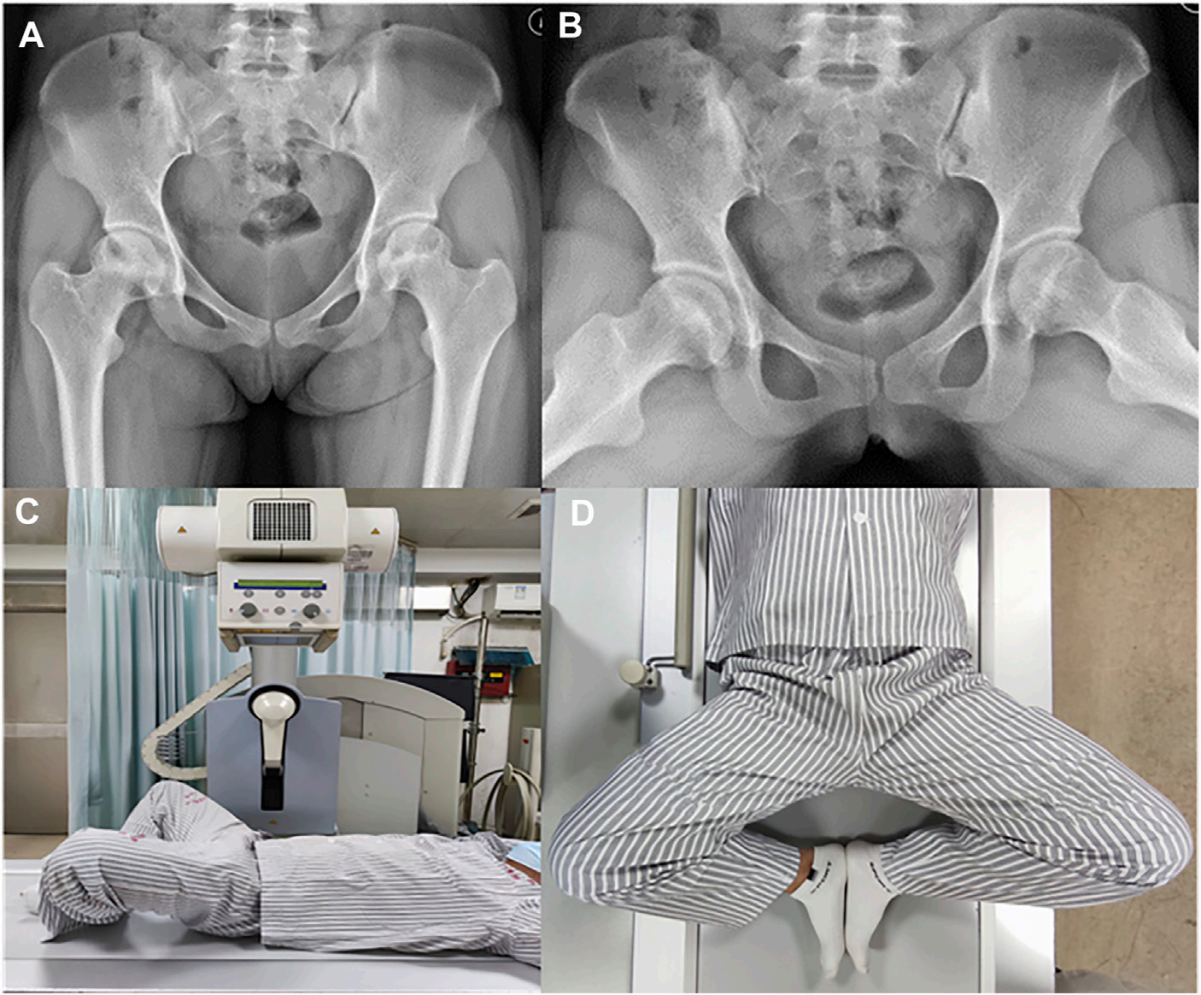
Radiological assessment (A) AP view of hip joint. Patients were positioned supine on the x-ray table and bilateral legs abducted in neutral position so that the distance between the two feet is equal to shoulder width. (B) FLL view of hip joint. (C) For the FLL x-rays, patients were positioned supine on the x-ray table and the bilateral hips were flexed at a degree of 30°. (D) For the FLL x-rays, the thigh was abducted and externally rotated while ensuring that the feet contacted close to each other at the level of ipsilateral knee. The x-ray beam was directed anterior to posterior and centered on the femoral head while the plane of the pelvis was parallel to the plane of the table (Wei QS. et al., 2019).
